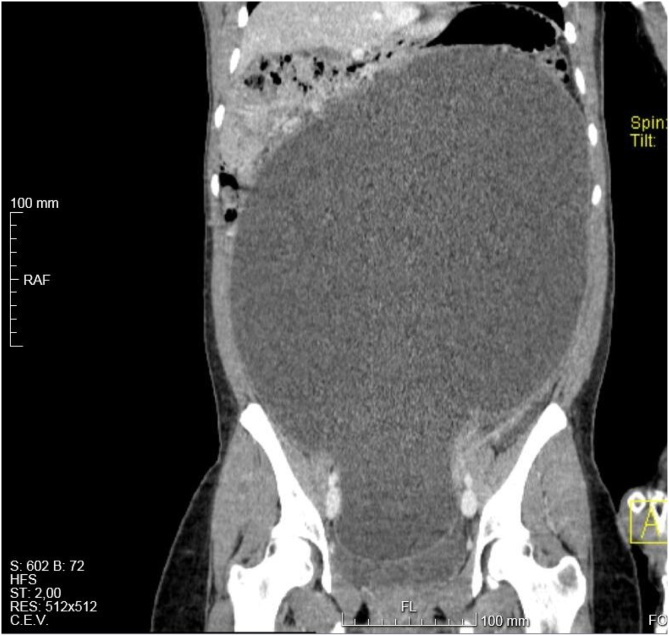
Coronal image of abdominal and pelvic CT showing the large size of the cyst and its effects on the surrounding structures.
